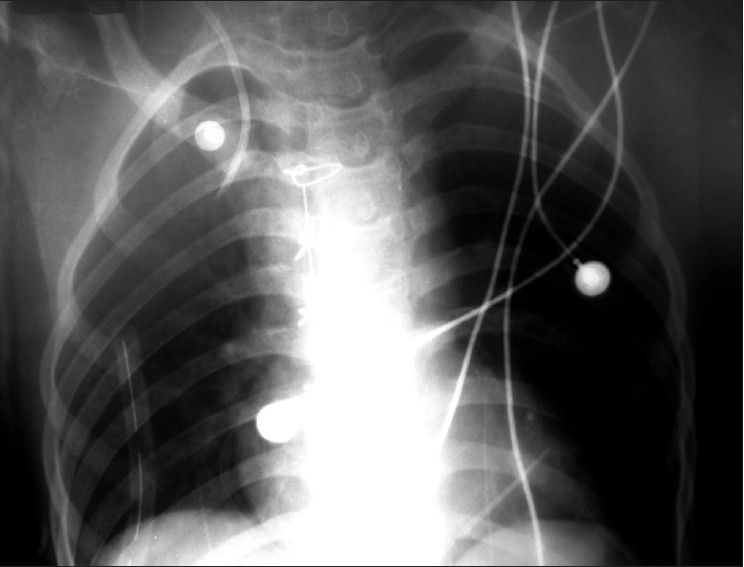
X-ray chest showing tip of the central venous catheter at apex of the right lung
